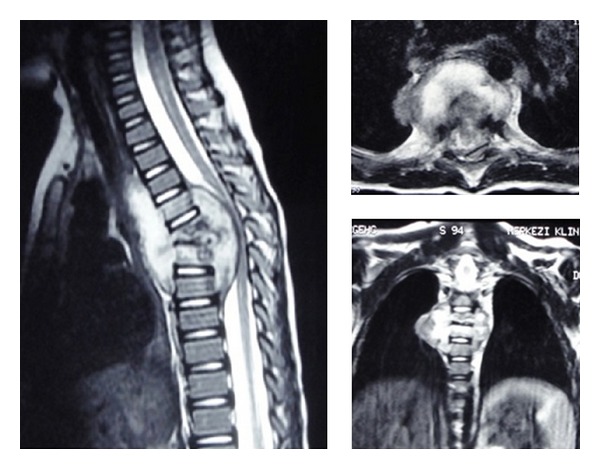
Preoperative MR images demonstrated a large paraspinal abscess.
